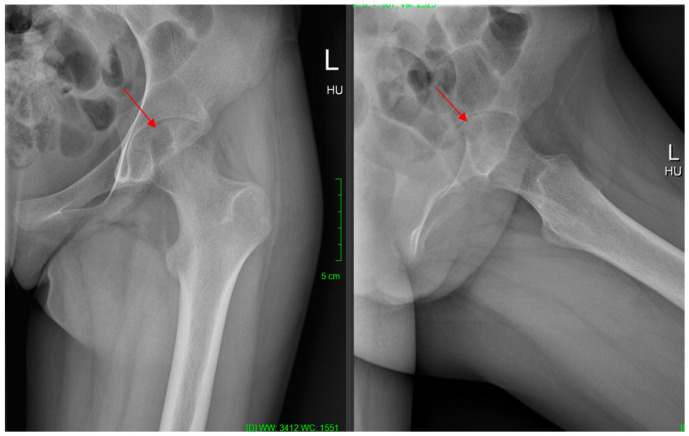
Preoperative 2-dimensional hip joint radiograph clearly showing the cystic lesion in the femoral head.
